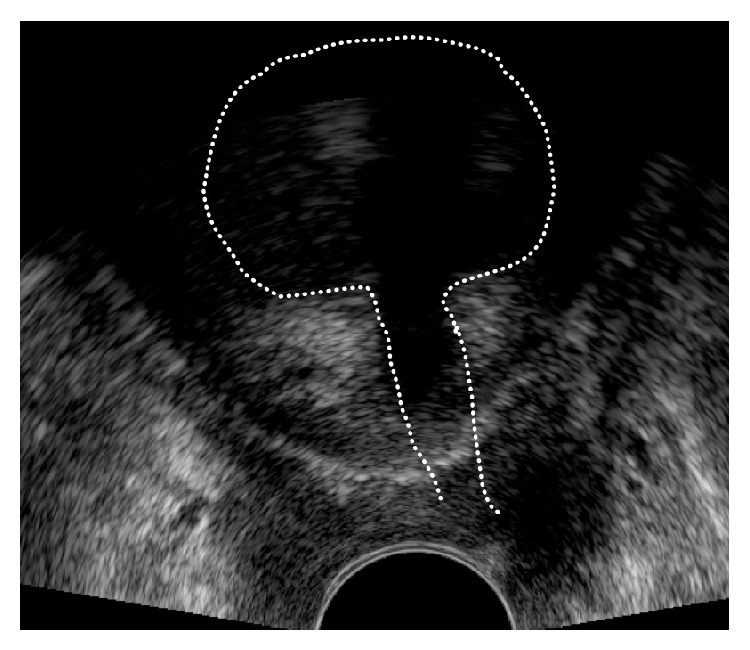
Pelvic floor ultrasound examination in frontal plane. Asymmetric sling position. On the right hand side of the picture the distance of the sling to the LSM complex is much shorter than on the left hand side. A loosening of the implant on the right hand side should be undertaken.
